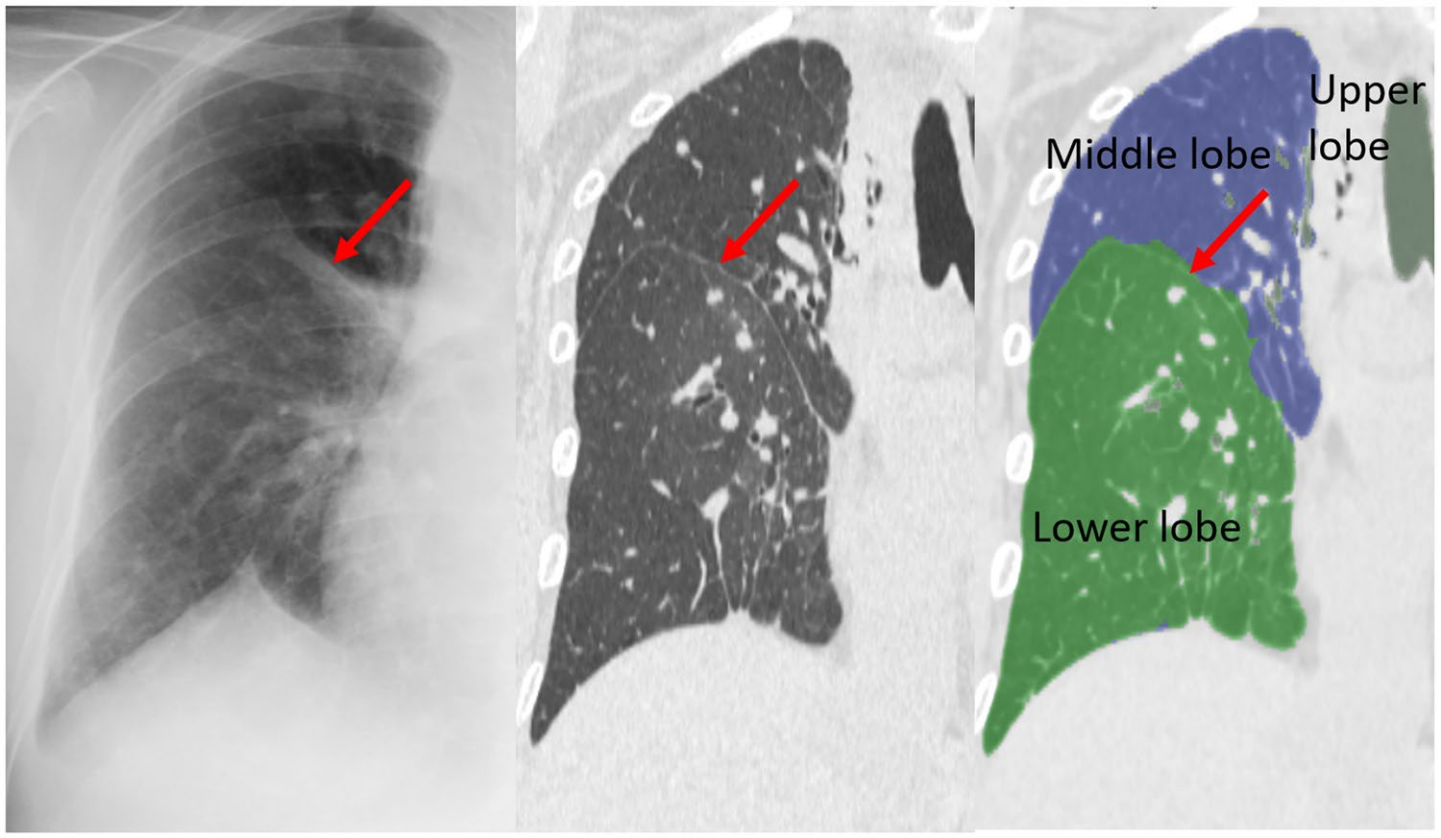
Case 8. Line 11. 51-year-old woman with gastric cancer. Chest X-ray shows the Kerley A-line in the right lung. The line corresponds to the deformed right major fissure on CT. The right upper lobe is complete atelectasis
